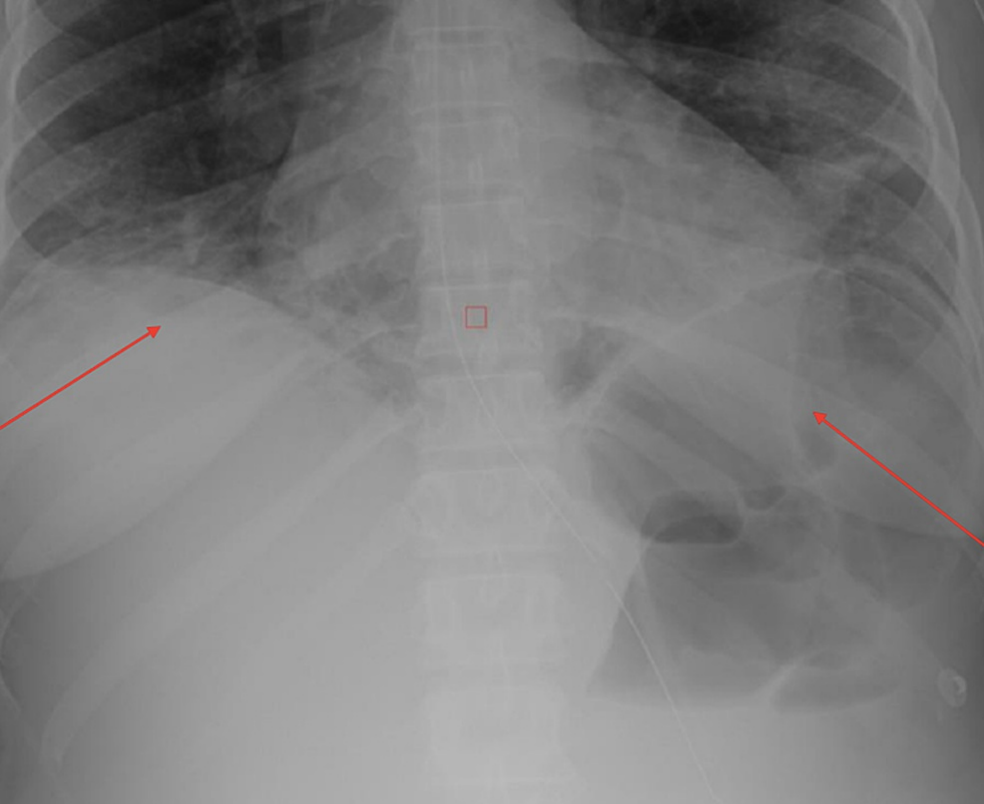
Chest x-ray of the patient indicating pneumonia or aspiration (red arrows)
